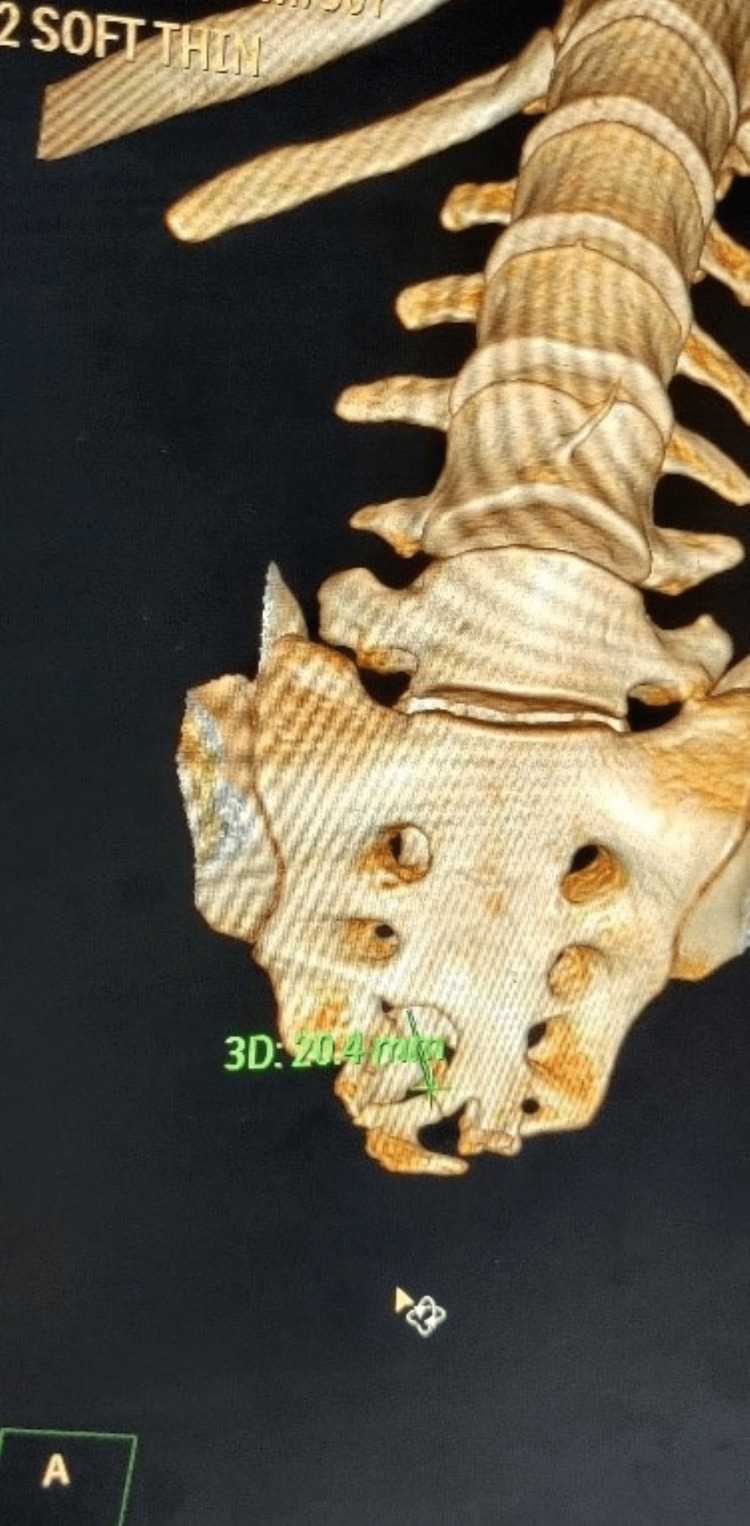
3D CT of sacrum showing defect in ventral part at S3 level
